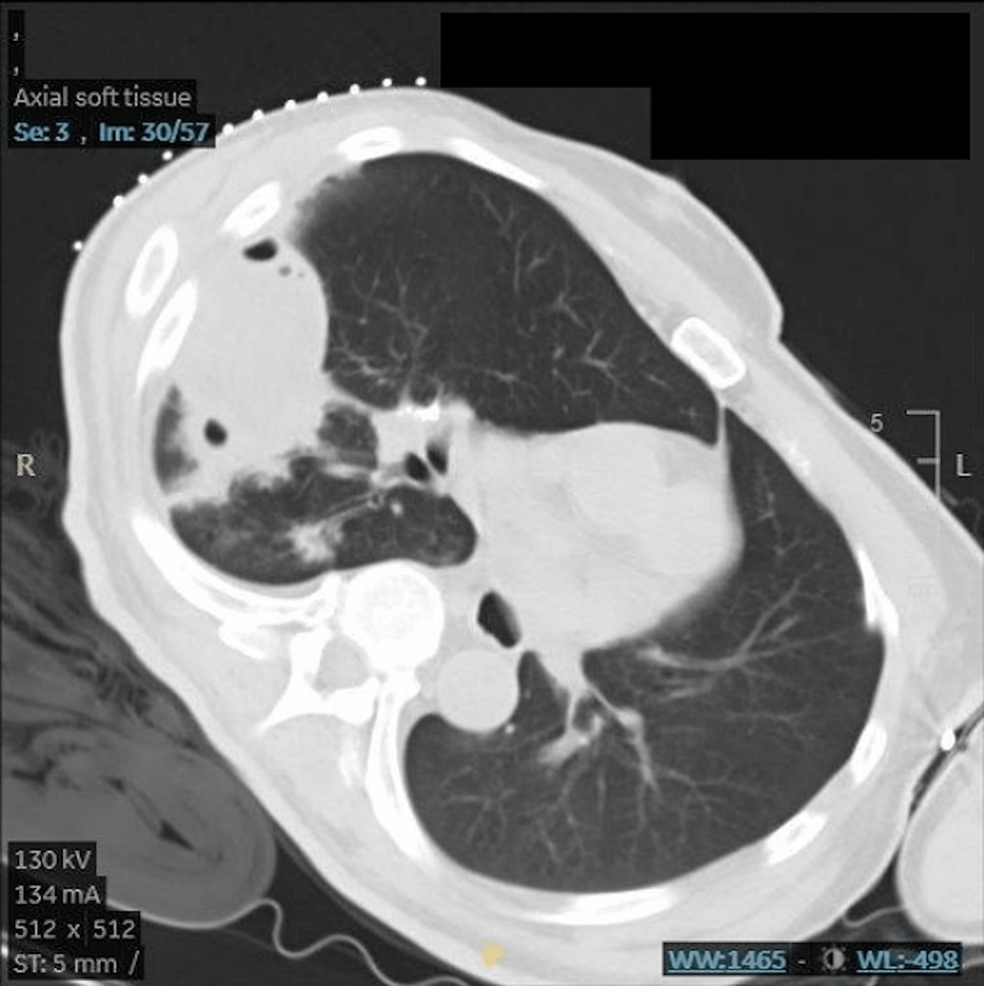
CT scan of the abscess prior to drainage
